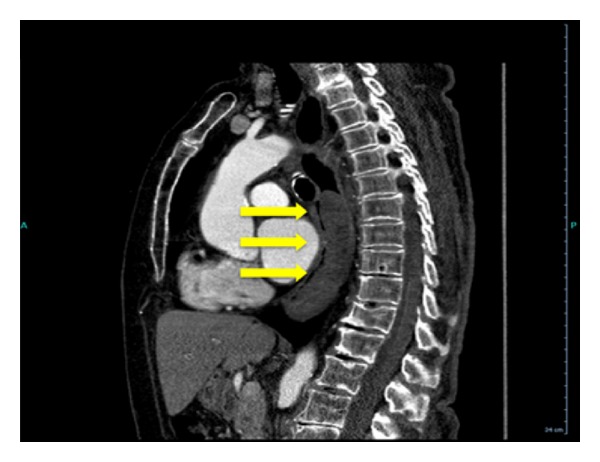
CT sagittal view of esophageal hematoma (arrows).
